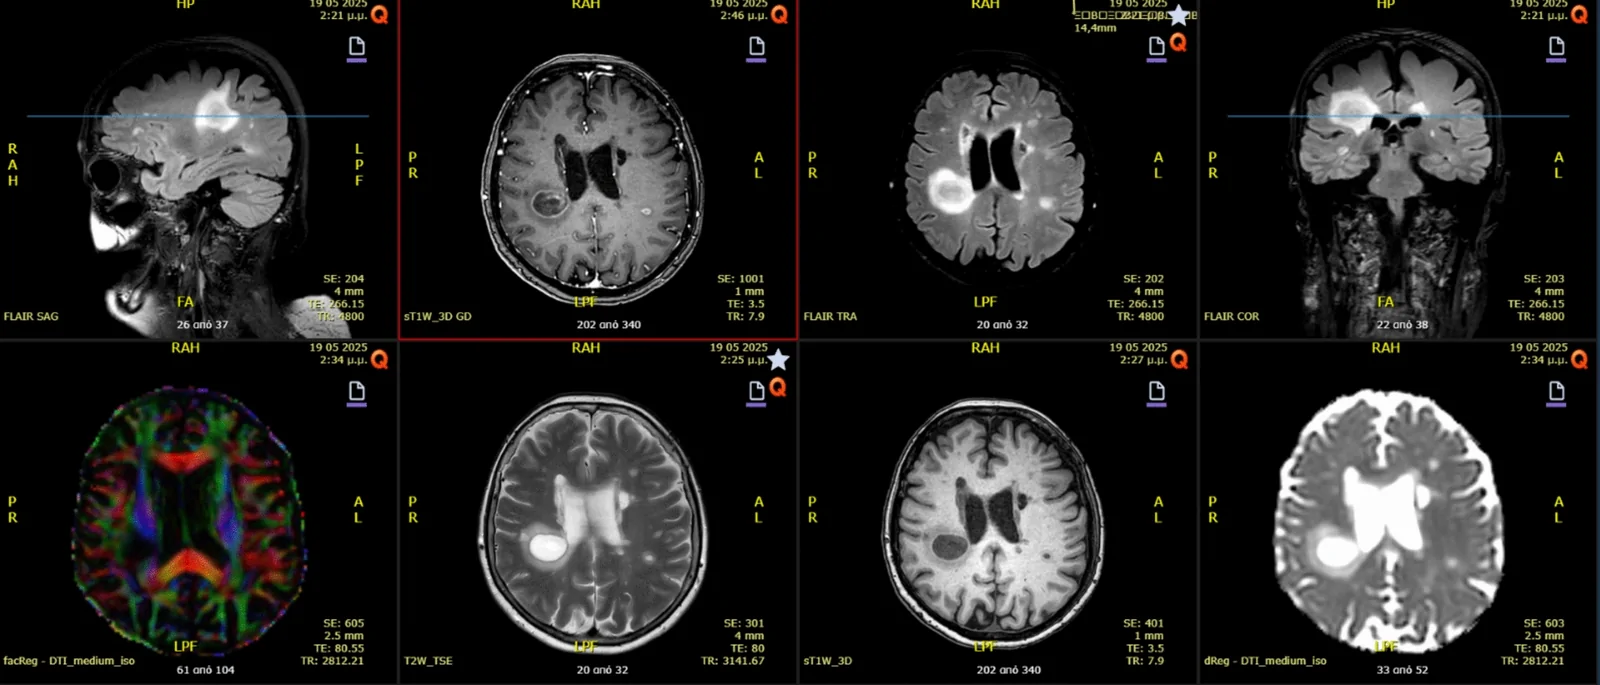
Brain MRI demonstrating tumefactive MS – Multiple hyperintense lesions on T2/FLAIR sequences with corresponding T1 hypointensity are visible in the periventricular and subcortical white matter. The dominant right parietal lesion (2.5 × 1.7 cm) exhibits a characteristic "double ring" appearance on FLAIR, with both peripheral and internal rings demonstrating enhancement following gadolinium administration. The lesion shows free diffusion on ADC maps with associated diffusion restriction in the enhancing rings. Perfusion imaging reveals no increased cerebral blood flow
